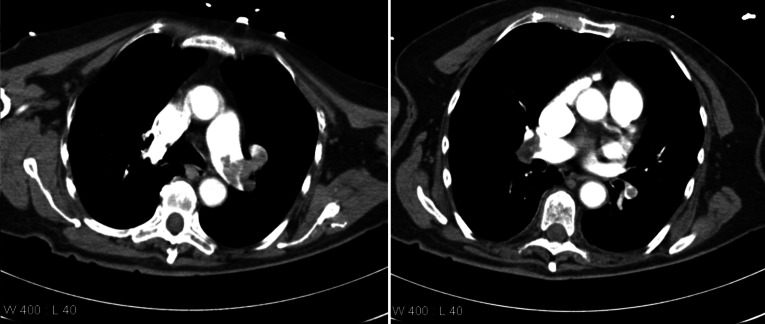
Case—thoracic computed tomography
